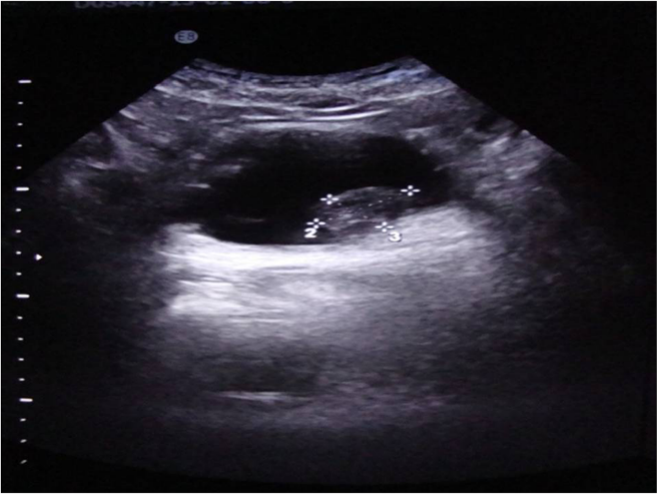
Echogenic ultrasound image at the left wall of the bladder.
